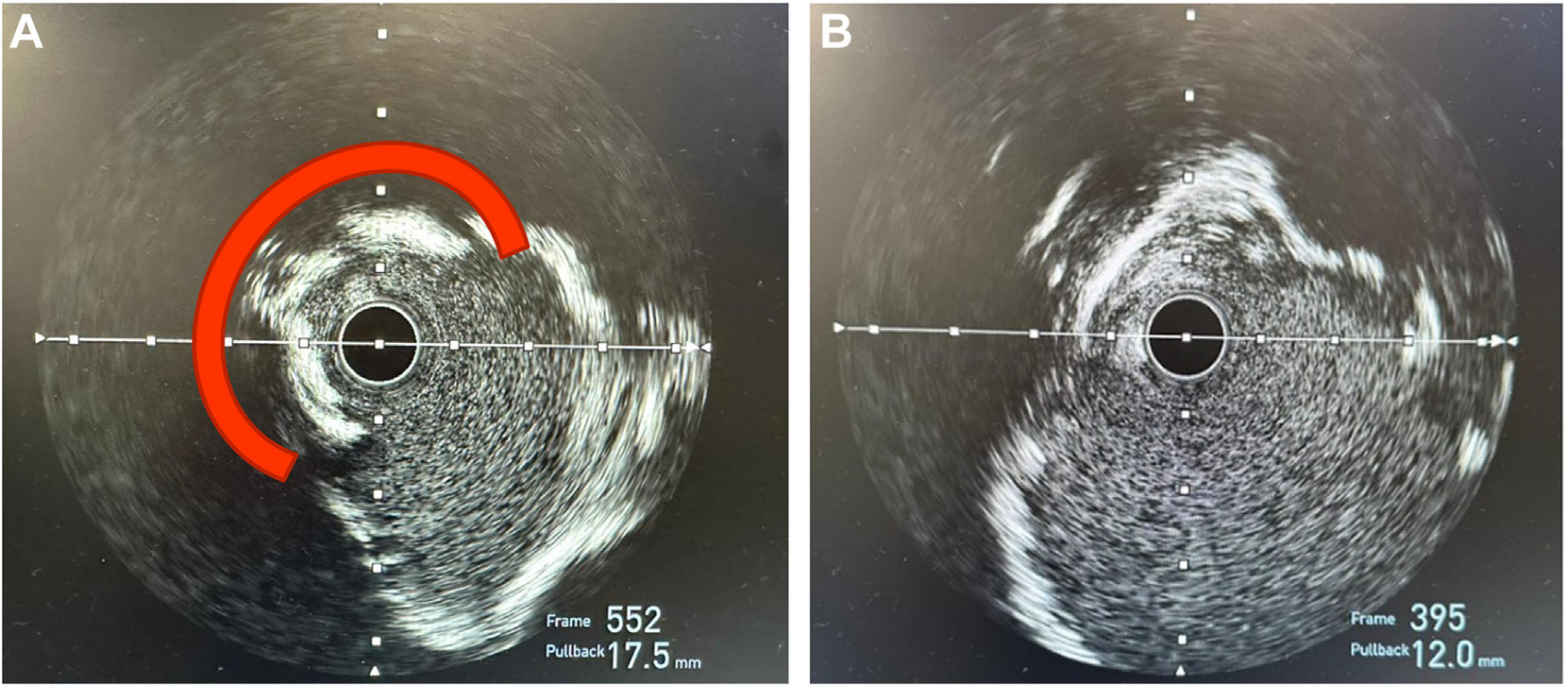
Intravascular ultrasound images. (A) Preinterventional intravascular ultrasound with a >180° arc of unfractured calcification (red). (B) Postinterventional intravascular ultrasound demonstrating significant improvement in-stent eccentricity.
